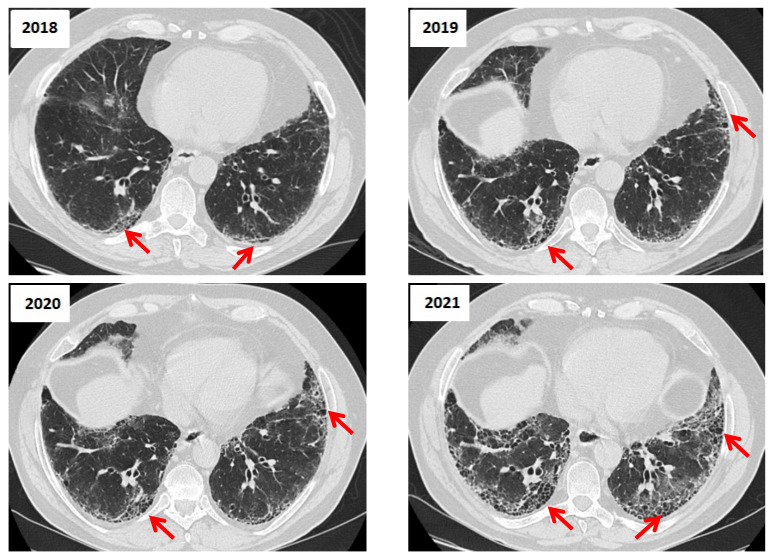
Longitudinal HRCT Changes of a UIP Pattern in a Patient With IPF (2018–2021). 2018: The baseline HRCT shows subpleural and basal predominant reticulation with early honeycombing and traction bronchiectasis, consistent with UIP. Ground-glass opacities are minimal (red arrows). 2019: There is a slight increase in reticular opacities and honeycombing, with more pronounced architectural distortion, suggesting disease progression (red arrows). 2020: Further fibrotic progression is evident, with an increase in honeycombing and traction bronchiectasis. The lung volume appears slightly reduced (red arrows). 2021: The final HRCT shows extensive fibrosis with coalescent honeycombing, more severe traction bronchiectasis and significant volume loss, indicative of advanced disease. Ground-glass opacities remain minimal, supporting a fibrotic rather than an inflammatory process (red arrows).
